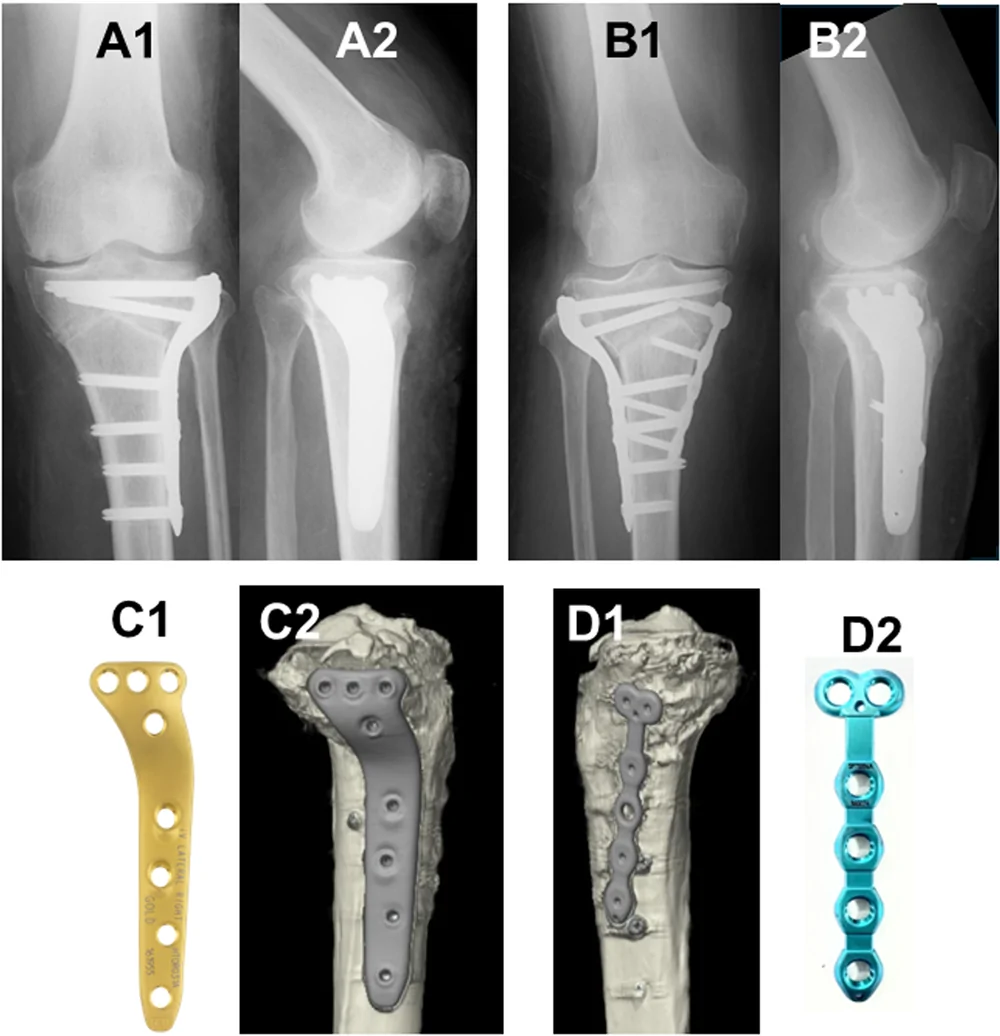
Postoperative radiographs of conventional single‐plating technique (a1, a2) and double‐plating technique (b1, b2). (c1, c2) Locking compression plate fixed with 5.5‐mm cannulated locking screws. (d1, d2) Small locking plate fixed with 4.2‐mm cannulated locking screws. (b1, b2, c2, d1) Images obtained from the same knee.
